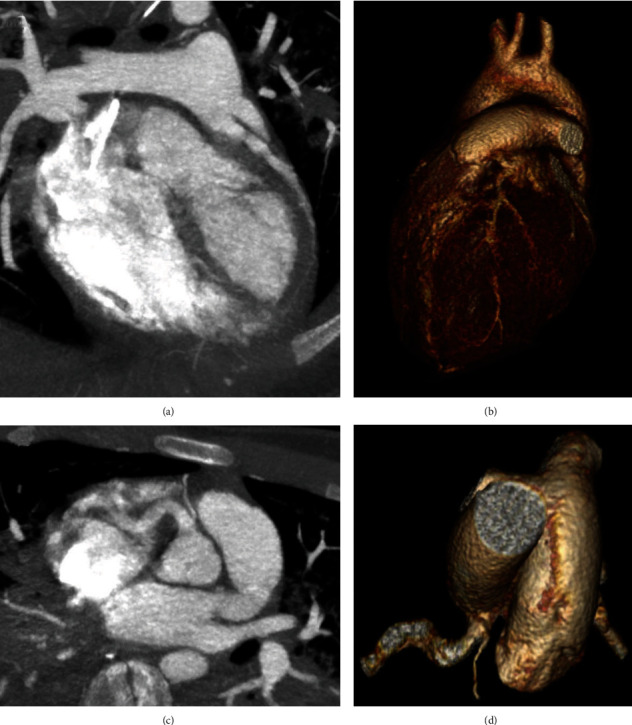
Computed tomography. Three-dimensional multiplanar and volume rendering reconstructions. (a, b) Left coronary artery connected to the pulmonary trunk; (c, d) right coronary artery (and its marginal branch) connected to the aorta.
